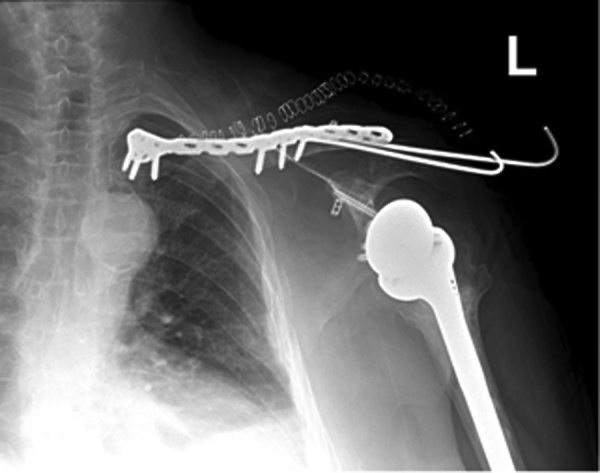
Post operative radiograph of the left shoulder showing plate and screws construct with two K-wires, showing satisfactory alignment of the bipolar clavicular fracture, along with an intact, stable reverse shoulder prosthesis.
